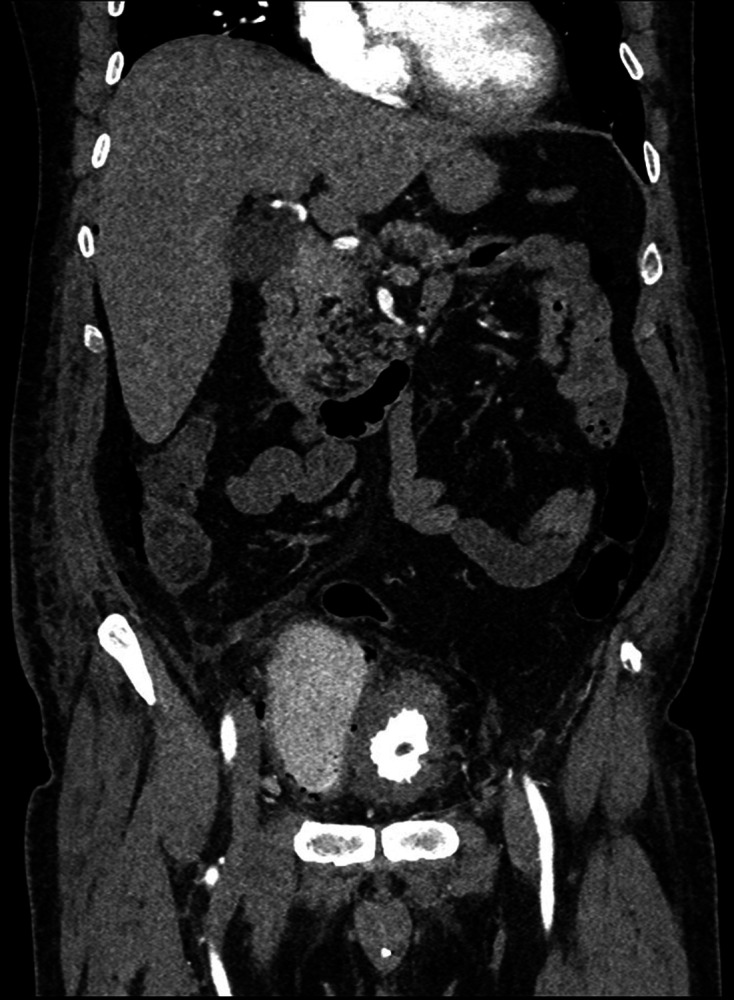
First coronal delayed phase CT (Day 3 of admission), on soft tissue window
